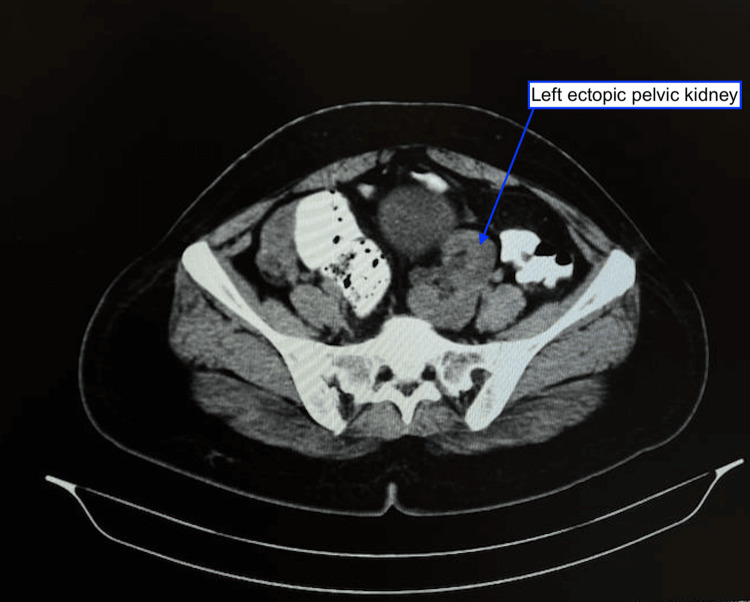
CECT of the abdomen and pelvis confirms the presence of a left ectopic kidney located in the left paramedian pelvic region, with the hilum facing anteromedially CECT: Contrast-enhanced computed tomography
